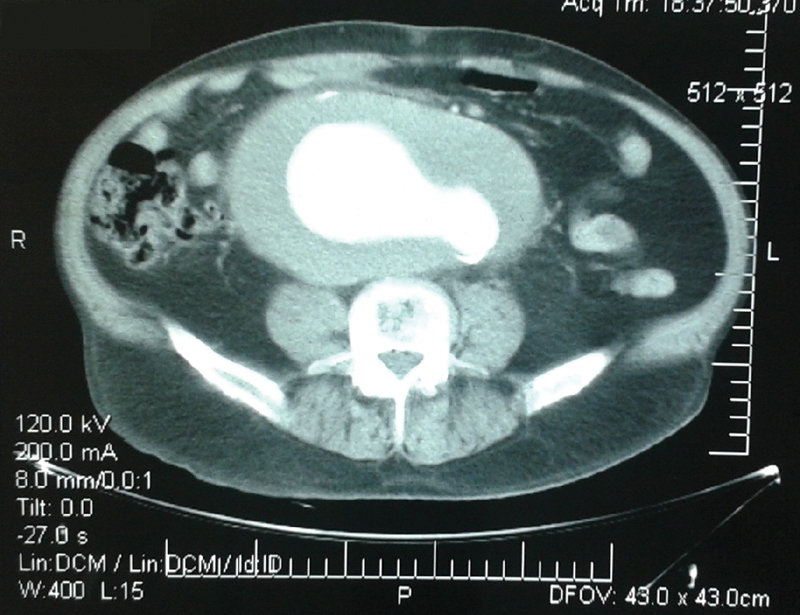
Contrast-enhanced computed tomography demonstrates a giant aortoiliac aneurysm at the level of the aortic bifurcation with extensive thrombus.
